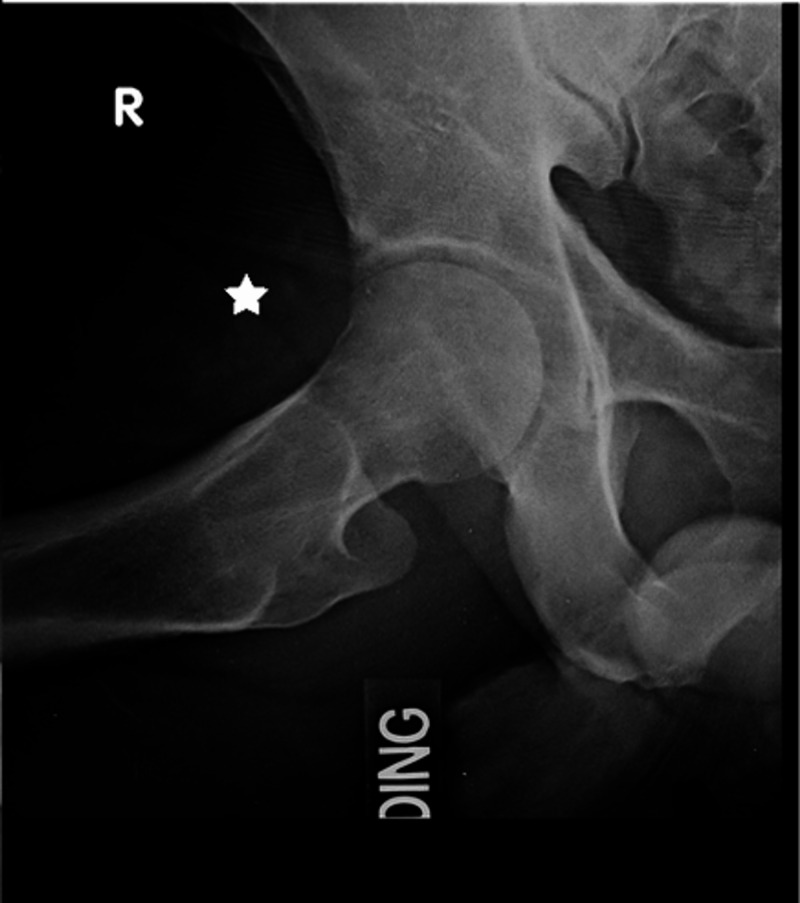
Post-Operative Radiograph Case 2 Modified Dunn radiograph taken 6 months postoperatively. The star indicates that there has been no recurrence of HO.
